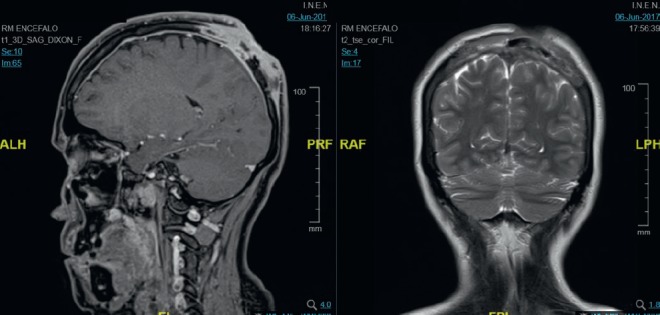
Brain MRI (06.06.17): Neoformative tissue, which infiltrates the bone marrow of the cranial calotte, in the right frontal bone, left occipital with bilateral parietal bone to left predominance, the latter infiltrates the adjacent soft parts, necrotic and ulcerated in the adjacent subcutaneous plane associated with osteolysis of the calotte, infiltrates the adjacent pachymeninges and the posterior aspect of the interhemispheric furrow, without infiltration of the parenchyma.
